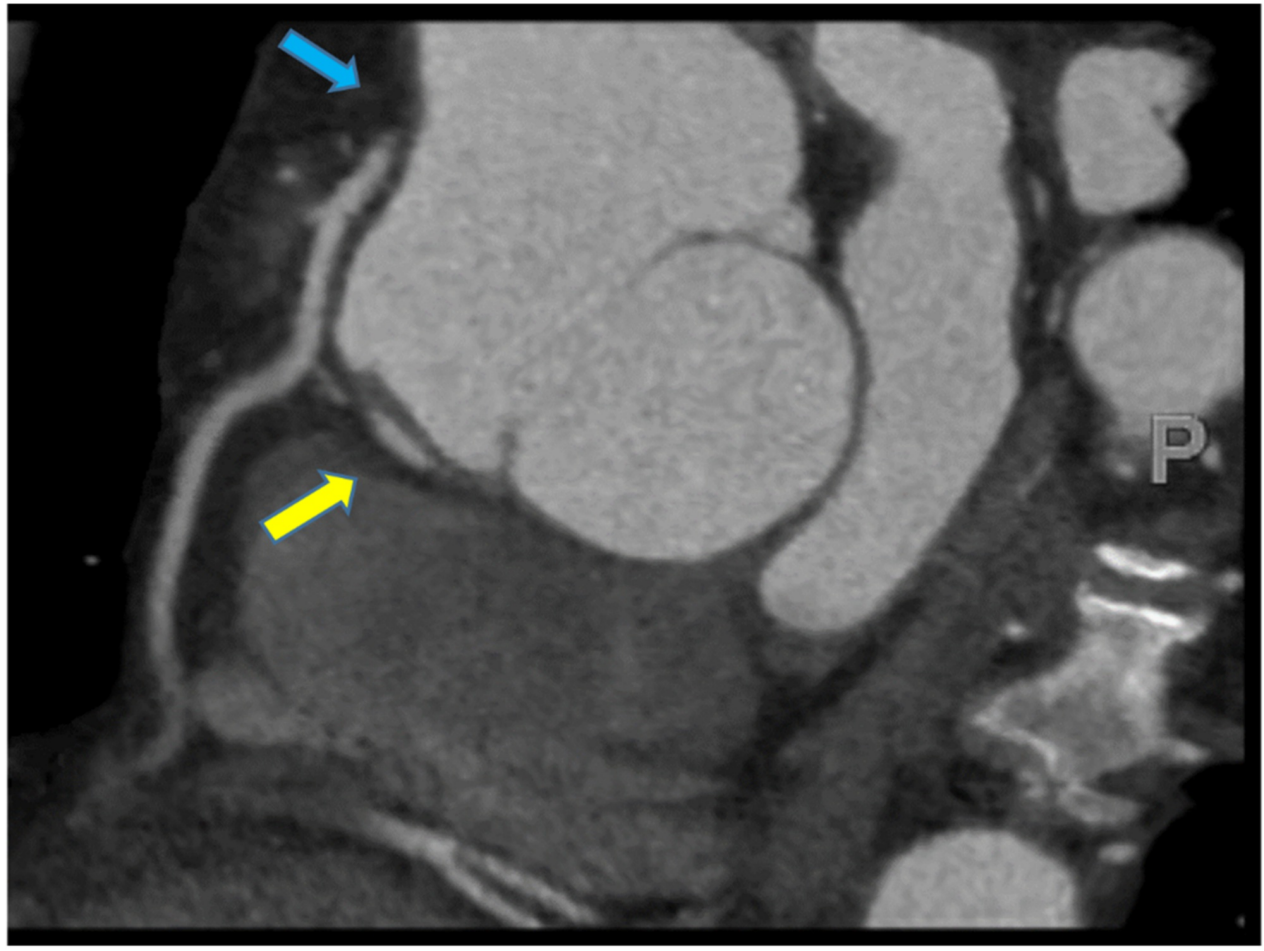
Cardiac computed tomography angiography showing the absence of communication between the ostium of the right coronary artery and the right sinus of Valsalva (blue arrow). Discrete transverse dissection flap visualized in the right sinus of Valsalva (yellow arrow).
